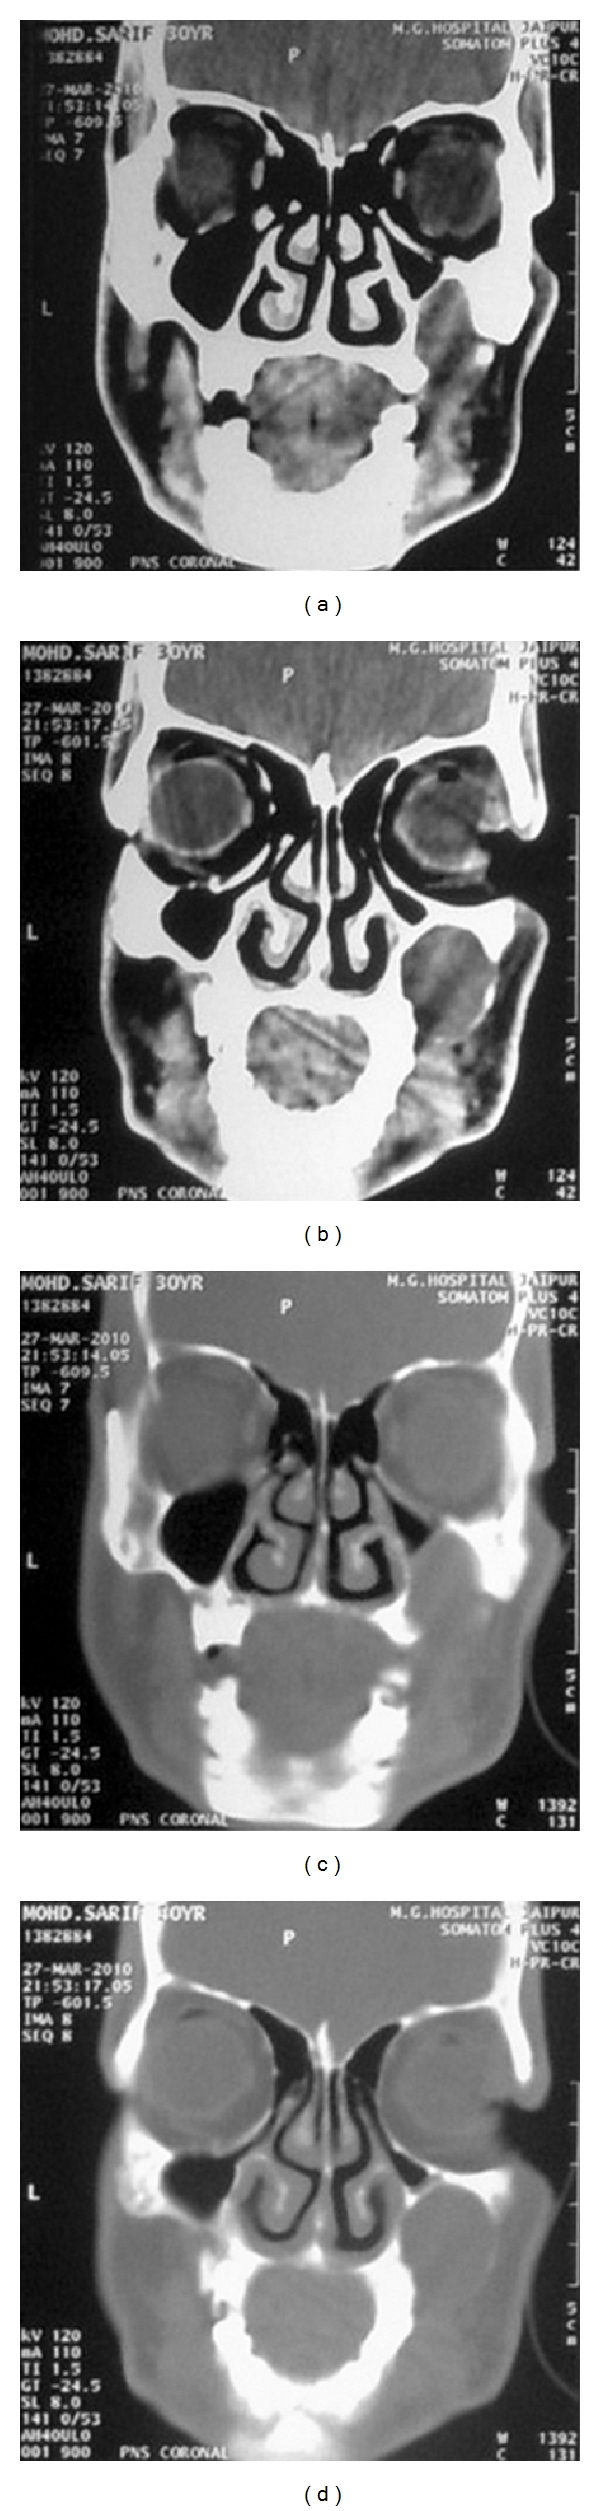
(a, b) CT scan view (coronal section soft tissue window), (c, d) CT scan view (coronal section bone window). Both views show a well defined soft tissue lesion causing displacement of inferolateral wall of the right sinus without involving it.
